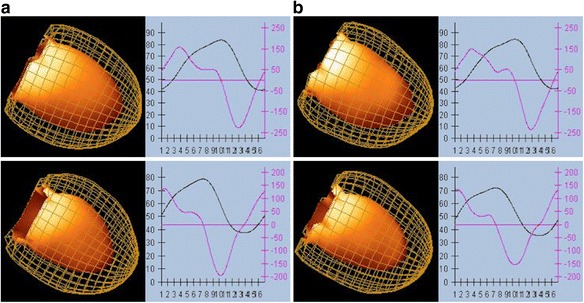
DNM 530c and DSPECT end-systolic volume rendering, volume (mL), and filling (mL/s). End-systolic volume rendering, volume (mL), and filling (mL/s) in single ^99m^Tc (a) and dual ^99m^Tc (b) condition using DNM 530c (*upper row*) and DSPECT (*lower row*)
